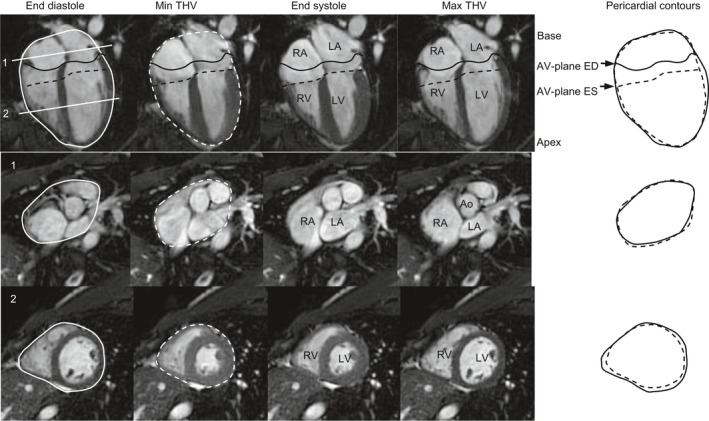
Inner and outer volume changes throughout the cardiac cycle. MR images of the subject in Fig 1 are shown in the following phases of the cardiac cycle: End diastole (ED), with closure of atrioventricular (AV) valves; Minimum total heart volume (Min THV), the lowest total heart volume; End systole (ES): conclusion of systolic ejection out of the heart through the aorta and pulmonary trunk; Maximum total heart volume (Max THV), the largest total heart volume corresponding to a time point just before atrial contraction. The figure shows the left ventricle (LV), right ventricle (RV), left atrium (LA), right atrium (RA) and aorta (Ao). The white lines 1 and 2 in the top left four‐chamber image indicate the positions of the short‐axis images in the numbered rows 1‐2. The black solid lines indicate the position of the atrioventricular (AV) valves in end diastole, and the black broken lines indicate the position of the AV valves in end systole. The solid circular contours denote the pericardial contour of the heart at ED. The broken circular contours denote the pericardial contour of the heart at the lowest total heart volume (THV). Note that the AV‐plane has not reached its most apical position at the time of minimum THV. End systole with the farthest excursion of the AV‐plane occurs ofter minimum THV.
